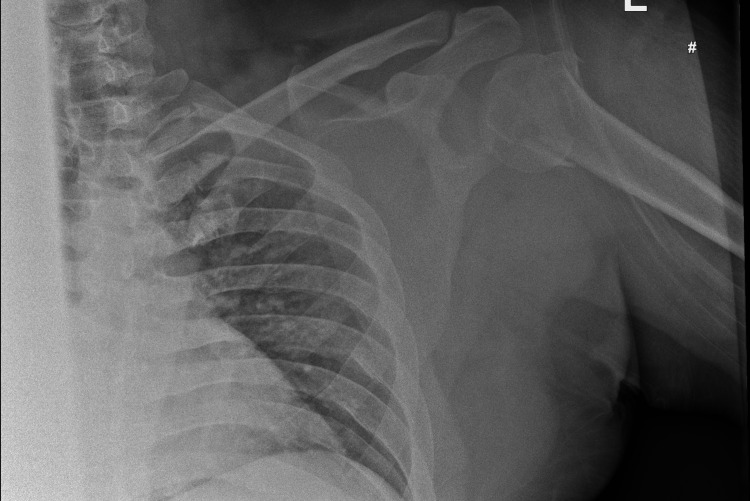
Anteroposterior radiograph of the left shoulder on presentation This demonstrates a varus-displaced comminuted proximal humerus fracture, but it does not clearly show the concomitant coracoid process and glenoid fractures.
